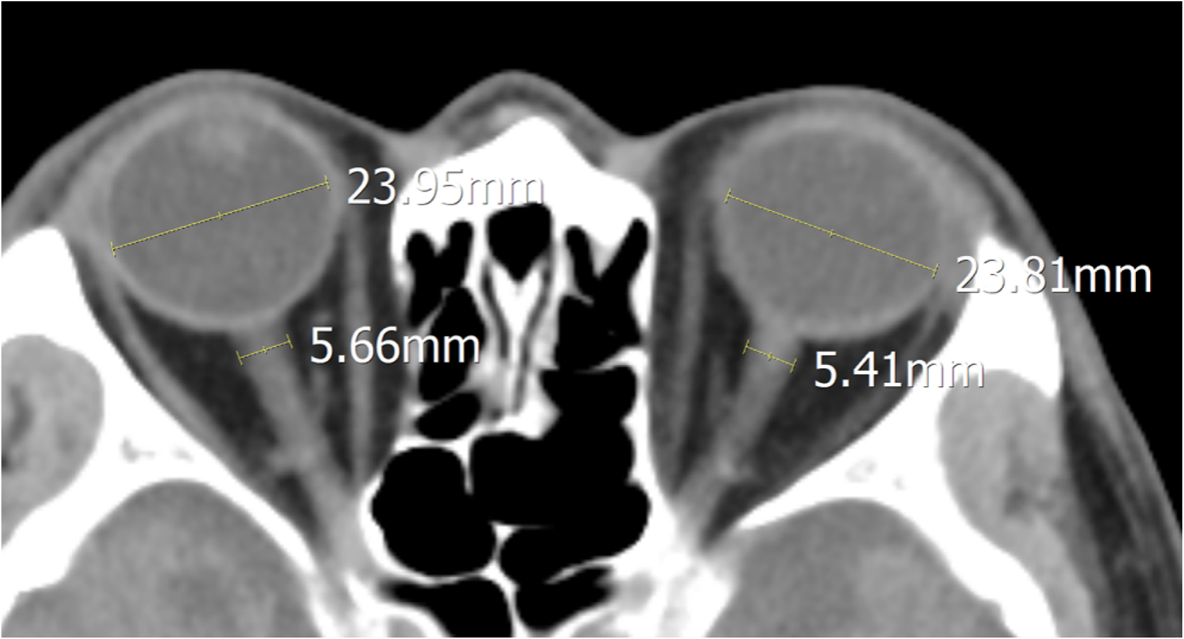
Computed tomography based measurement of ONSD and ETD. The ONSD was measured at a distance of 3 mm behind the eyeball, immediately below the sclera in a perpendicular vector with reference to the linear axis of the nerve. The ETD was defined as the maximal transverse diameter of the eyeball measured from one side of the retina to the other. ONSD, optic nerve sheath diameter; ETD, eyeball transverse diameter
